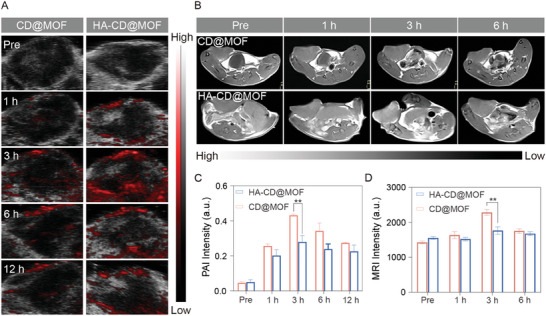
PAI/MRI ability of HA‐CD@MOF NPs. A) PAI images of the tumor after treatment with CD@MOF NPs and HA‐CD@MOF NPs. B) T_1_‐weighted images of the tumor at various time points after intravenous injection of CD@MOF NPs and HA‐CD@MOF NPs. C) Quantitative analysis of PAI intensity of the tumor; *n* = 3. D) T1‐weighted MRI signal intensity of the tumor; *n* = 3. Results are presented as means ± SD. p* < 0.05, *p* < 0.01, p* < 0.001, *p* < 0.0001.
